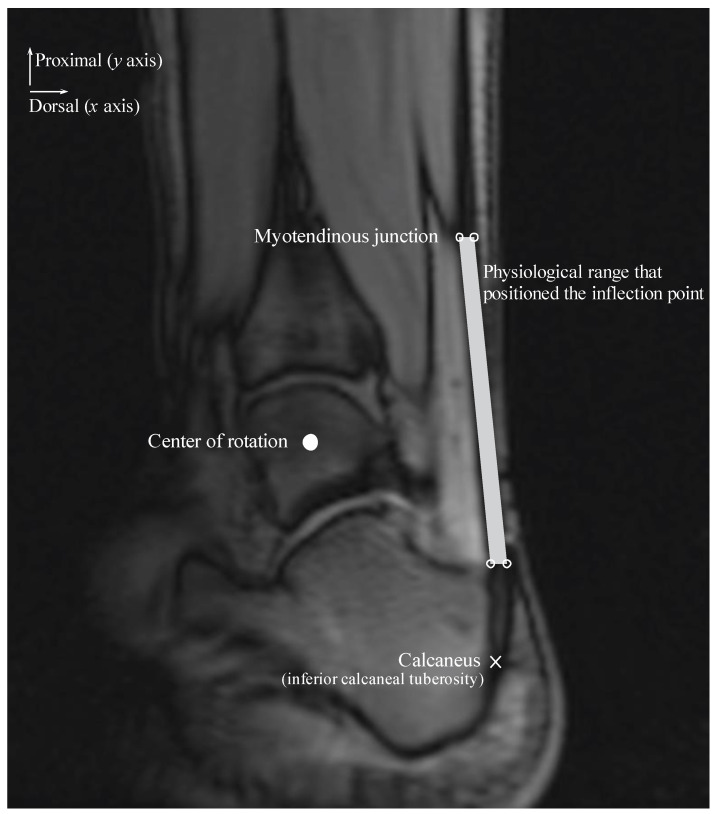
Representation of the physiological range of the inflection point within the Achilles tendon where the gain_AT_ is calculated. The positions of the start (i.e., myotendinous junction) and end (i.e., inferior calcaneal tuberosity) points of the Achilles tendon, and ankle center of rotation are shown in a typical sagittal-plane MR image from one subject. The ankle angle was set at approximately –10° (ankle dorsiflexed position). The physiological range of the inflection point is defined as the area within the Achilles tendon and is represented as the area (gray color) of a quadrilateral comprising four vertices (points; indicated by white circle symbols).
